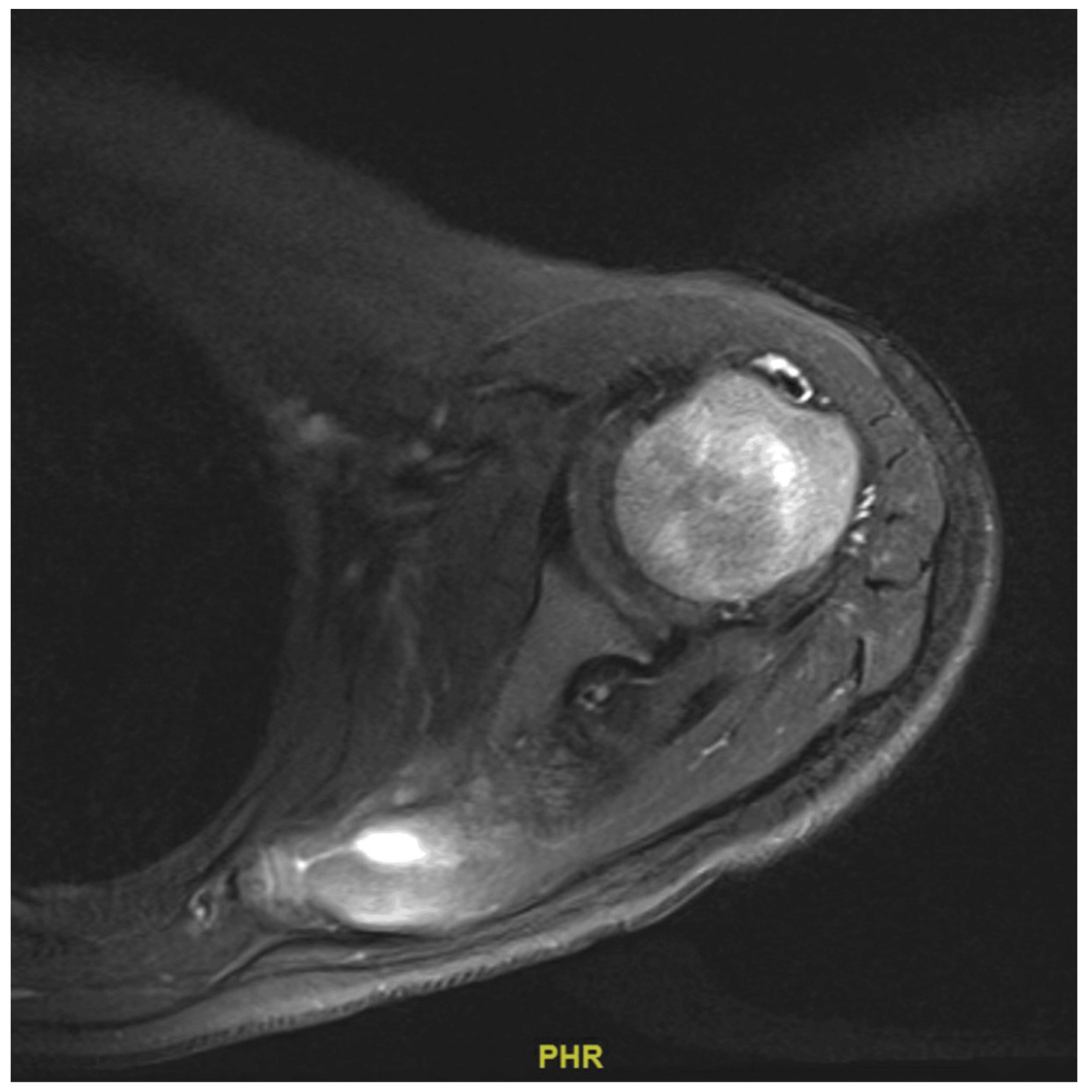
Post-chemotherapy magnetic resonance imaging scan showing residual lesion along the medial aspect of the mid left scapula
